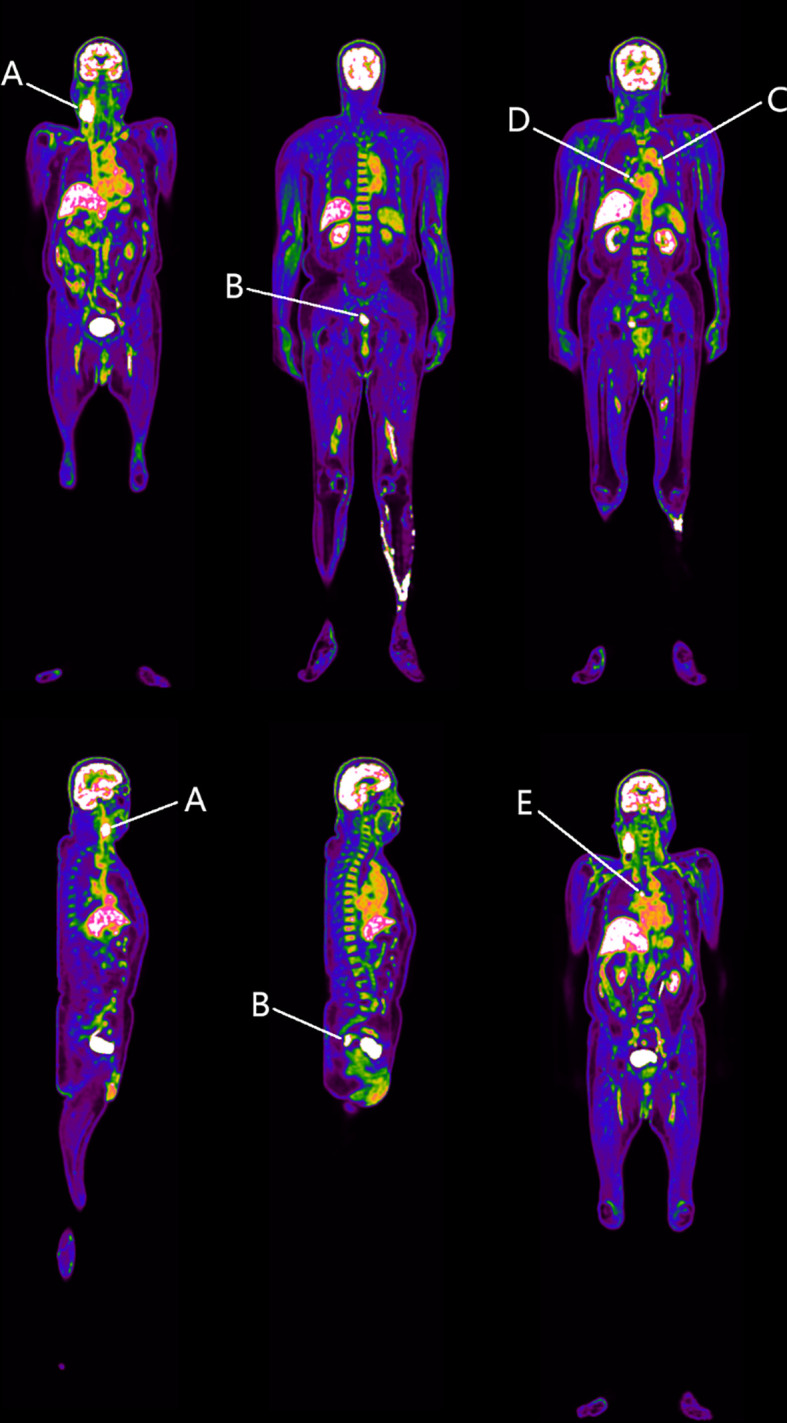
Identified regions with increased 18F-FDG uptake. Region A represents the right neck space-occupying lesion. Region B resides in the rectosigmoid junction. Regions C–E denote the mediastinal lymph nodes. At this stage, we speculate that Region B is the possible primary tumor responsible for Region A.
